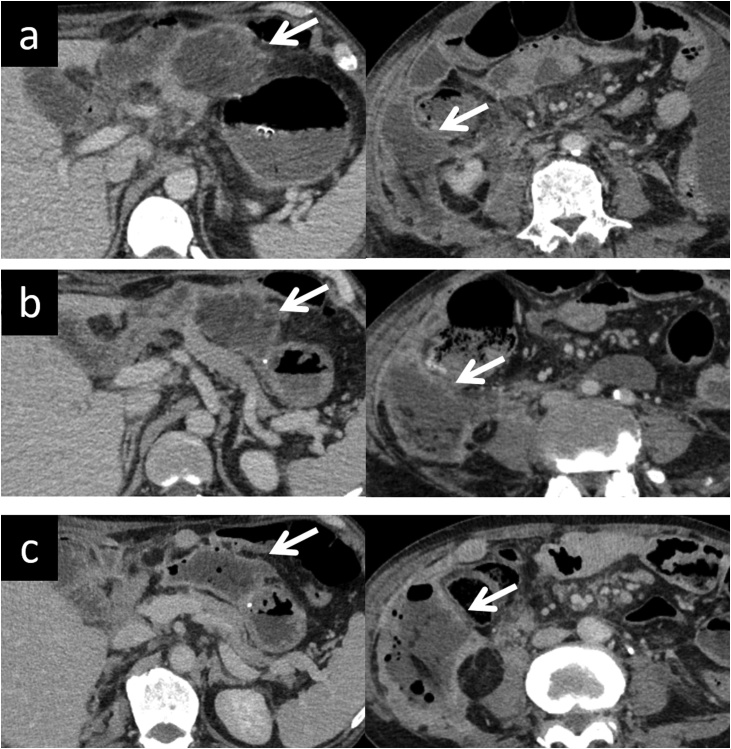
Abdominal CT scan obtained on (a)day16, (b)day23, and (c)day42 of hospitalization showing gradual transformation of ascites into an abdominal abscess (arrow).
